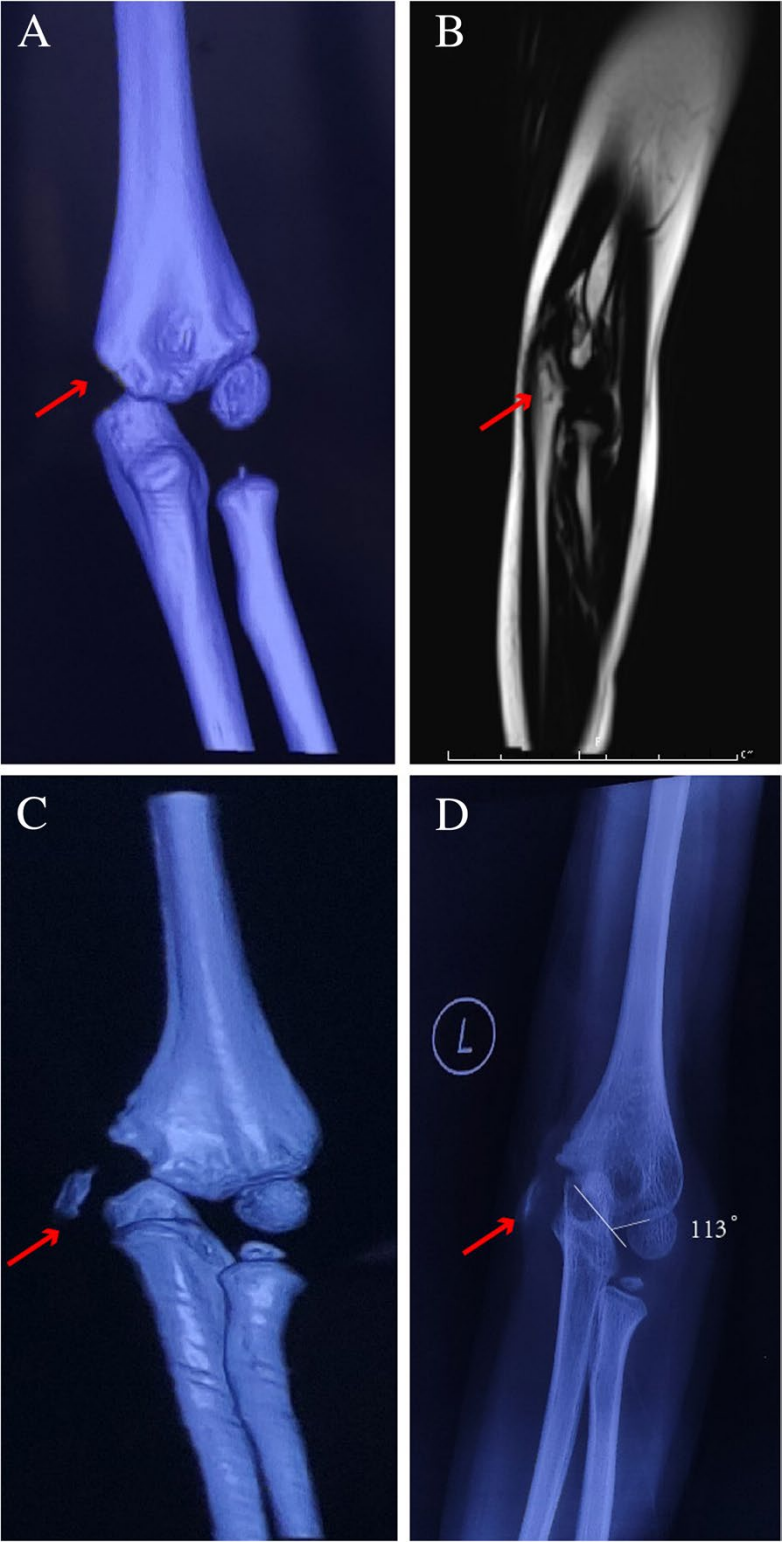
Imaging features of patients. A 3D-CT of the elbow 1 year ago, no abnormality was seen. B This MRI showed poor joint alignment and joint effusion. C Falling debris is clearly visible in the 3D reconstructed image. D X-ray examination can clearly show that the bone cortex is discontinuous and the broken ends are dislocated
